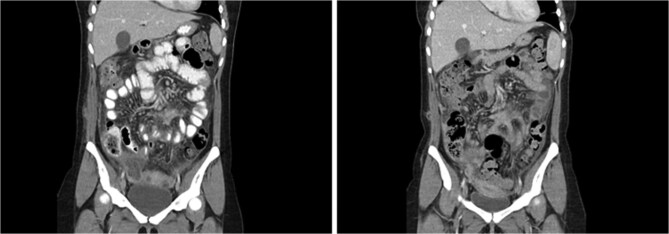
Coronal CT abdomen from admission 3 (left) and admission 4 (right). Heterogenous collection in right pouch of Douglas, progression of mesenteric/omental fat stranding, thickened loops of small bowel.
